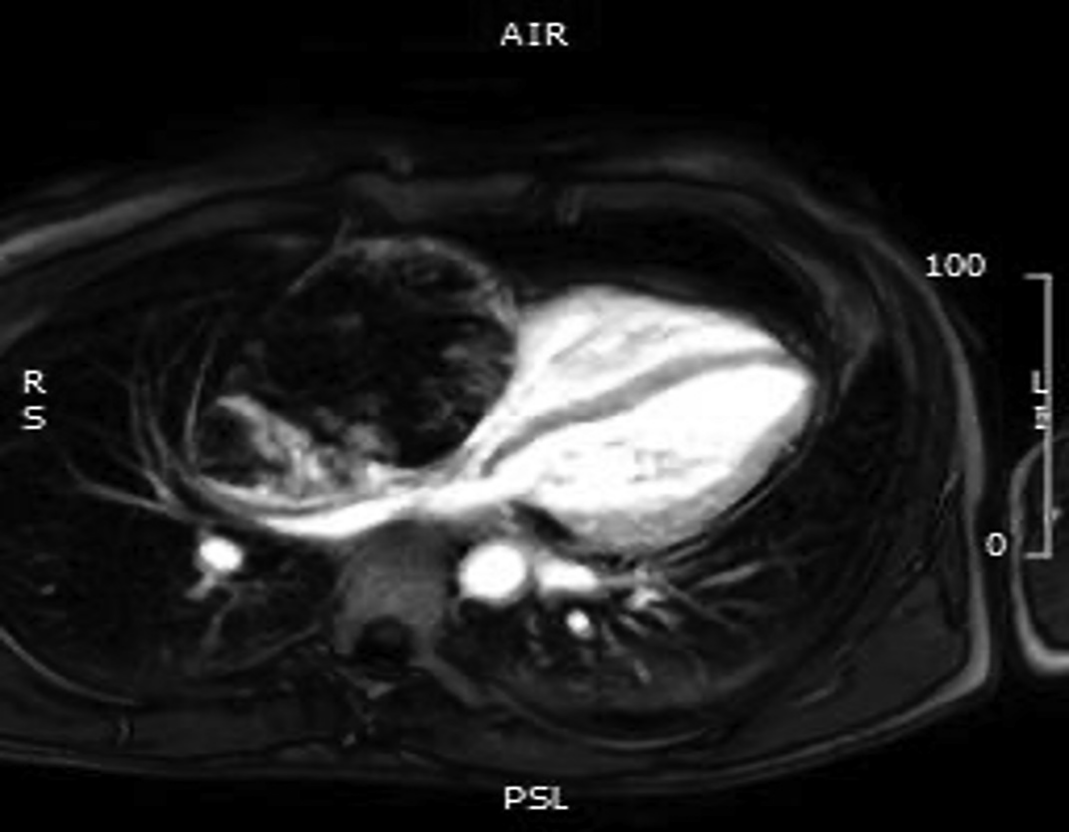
Early Gadolinium Enhanced Cardiac Magnetic Resonance Image of the Right Atrial Mass
